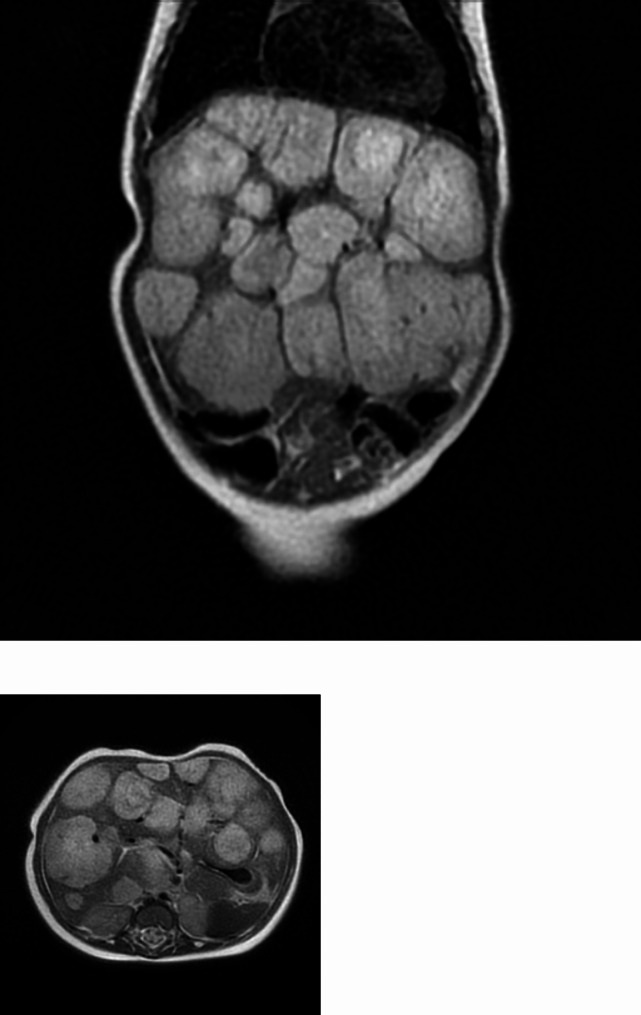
MRI of abdomen at the diagnosis of diffuse infantile hepatic haemangioma
